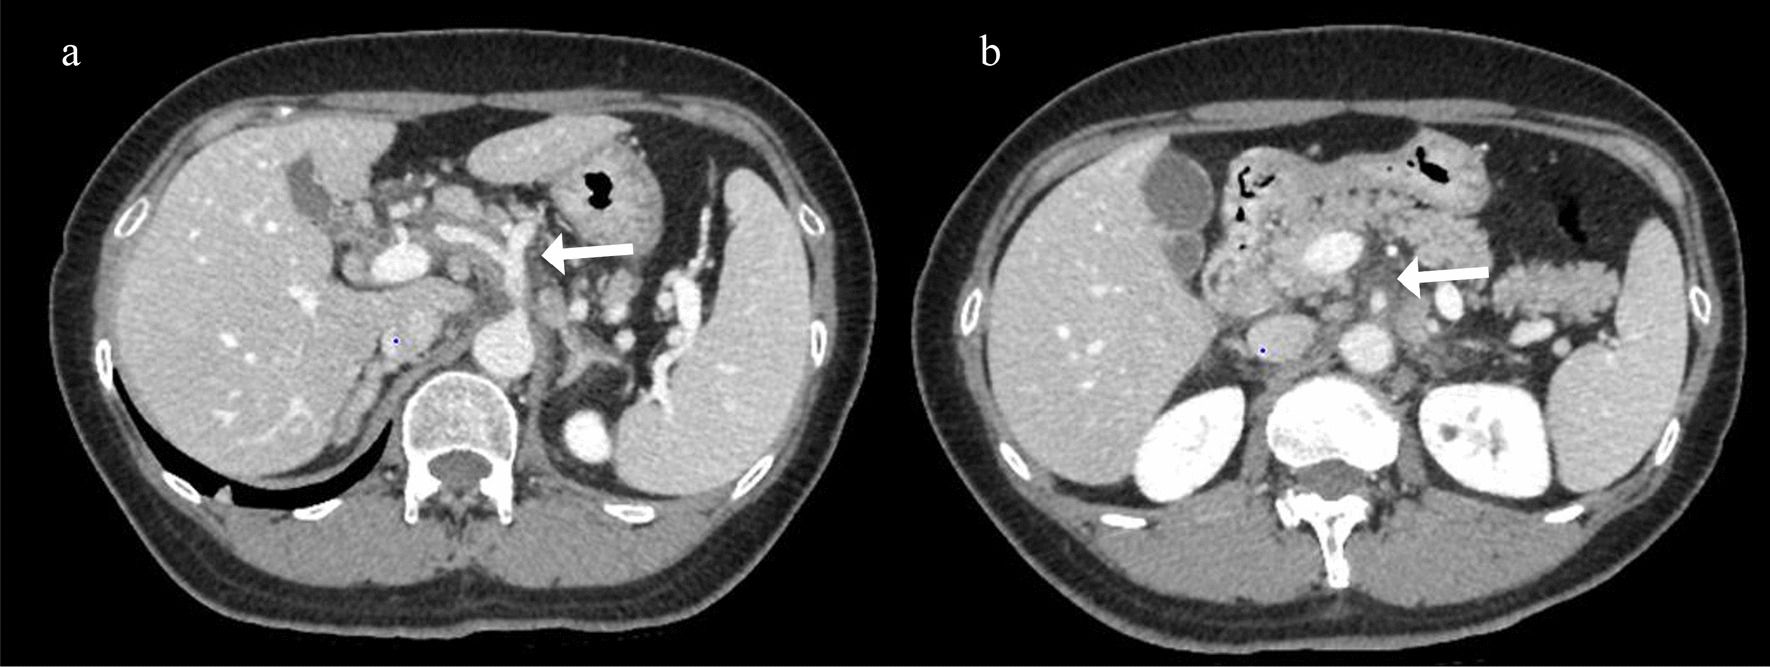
Abdominal computed tomography (CT) scan at the time of emergency department visit; a Increased CT value (fat stranding) around the celiac artery and its branches. b Fat stranding around the superior mesenteric artery
